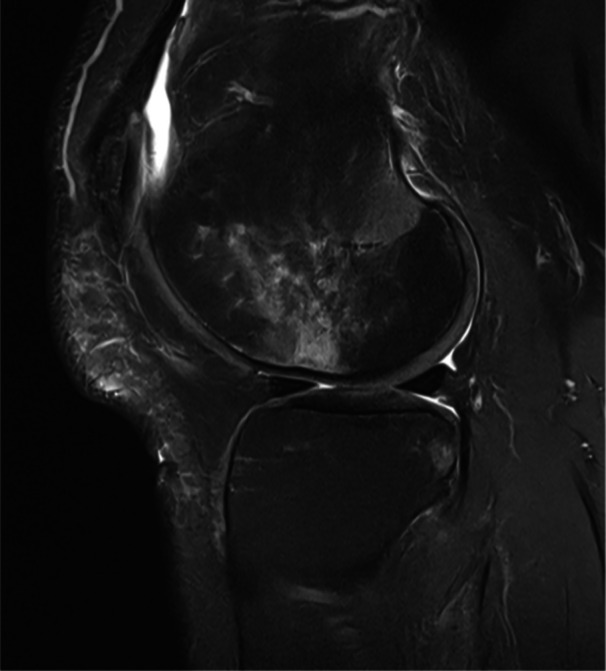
Magnetic resonance imaging (MRI—T2, sagittal) of a local bone marrow oedema at the right lateral femoral condyle of a 52‐year‐old patient with knee pain for 8 weeks and multilocular bone marrow oedema without trauma. Serological analyses revealed a disturbed lipid metabolism with an increase in lipoprotein (a). This patient may benefit from treatment with anticoagulants as a supplement to conservative physical therapy modalities.
